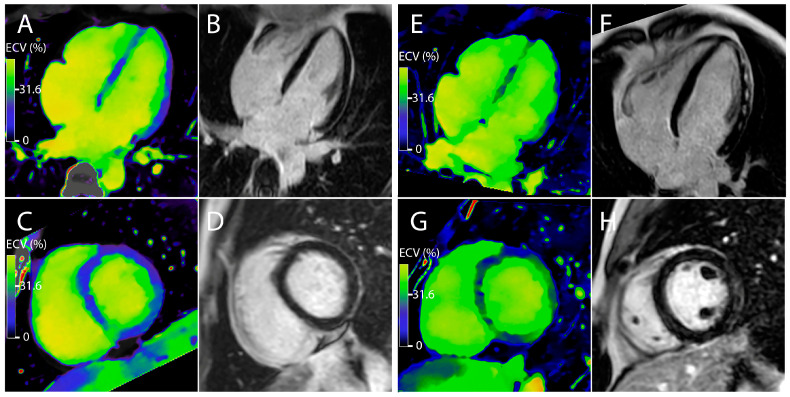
Representative cases of patients with suspected acute myocarditis. Left panel (A–D). Case of a 42-year-old woman with elevated high-sensitivity troponins at 83 ng/L at admission. A late phase DECT was performed at admission. The global ECV was measured at 29.7%. MRI did not show any late gadolinium enhancement. Right panel (E–H). Case of a 27-year-old man with elevated high-sensitivity troponins at 8000 ng/L at admission. A late phase DECT was performed at admission. The global ECV was measured at 35.1%. MRI showed multiple late gadolinium enhancement of the sub-epicardial myocardial wall in favor of myocarditis.
